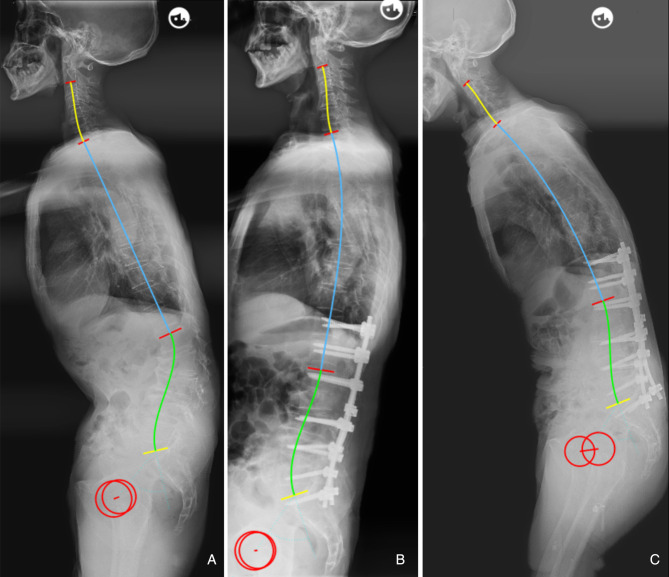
65-year-old male (A) preoperative sagittal standing full spine X-rays, pelvic incidence (PI) = 53.6°, thoracic kyphosis (TK) = 2.4°, lumbar lordosis (LL) = 11.5°. (B) immediate postoperative X-rays, PI = 53.3°, TK = 15.5°, LL = −26.3°; ideal LL = 0.7*53.3 + 0.4*15.5 + 1 = −44.5°, ΔLL = 26.3–44.5 = 18.2°. (C) Distal screw pull-out at 2.5-year follow-up.
